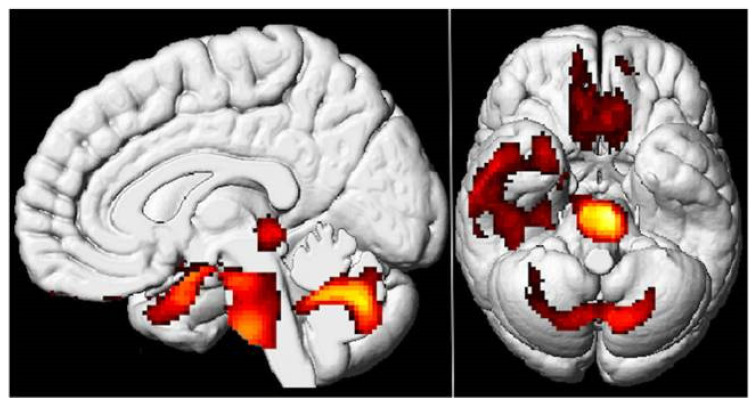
The 18F-FDG PET demonstrating hypometabolism in the bilateral rectal/orbital gyrus, the right temporal lobe, the bilateral pons/medulla brainstem, and the bilateral cerebellum in patients with PACS. Reproduced with permission from reference Guedj et al. [72].
